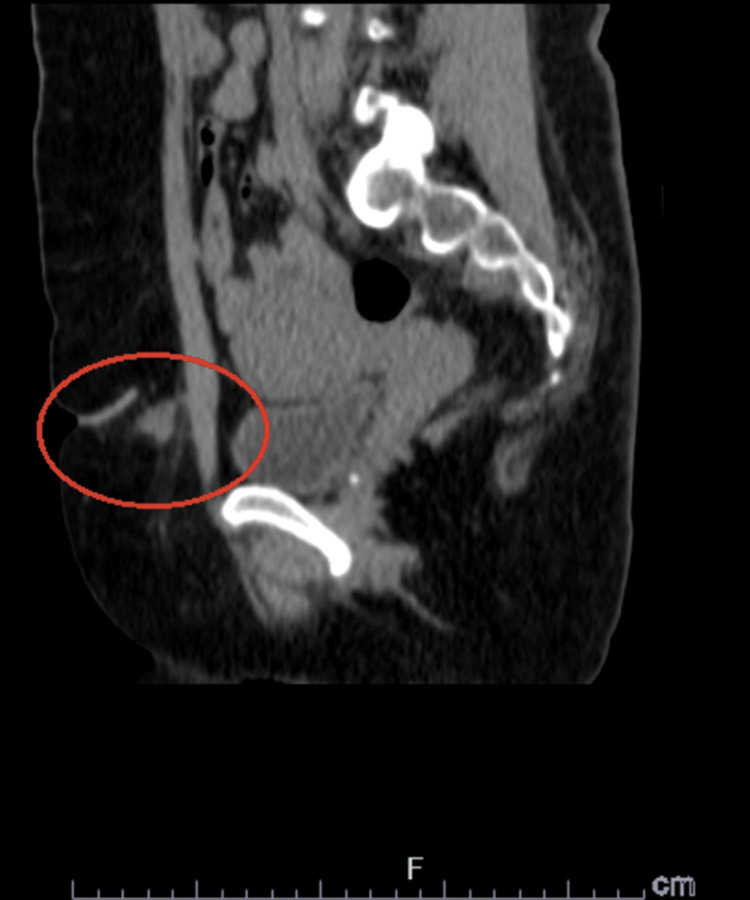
Sagittal CT abdomen view of the incidental 1.8 cm nodule. The nodule is well-circumscribed with homogeneous soft-tissue density and margins.
